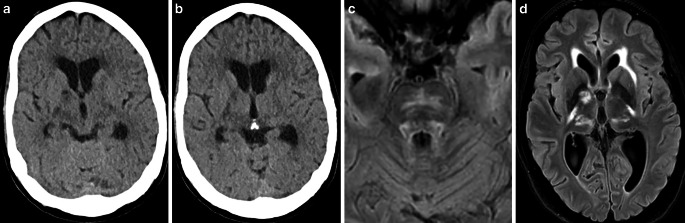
CT and MRI scans of a 57-year-old female presenting with confusion, speech disorder, bradykinesia, and mild right ptosis. Native CT images (axial views; a, b) and MRI with FLAIR images (axial views; c, d) demonstrated new lesions in the bilateral thalamus, bilateral internal capsule, left external capsule, and pons
